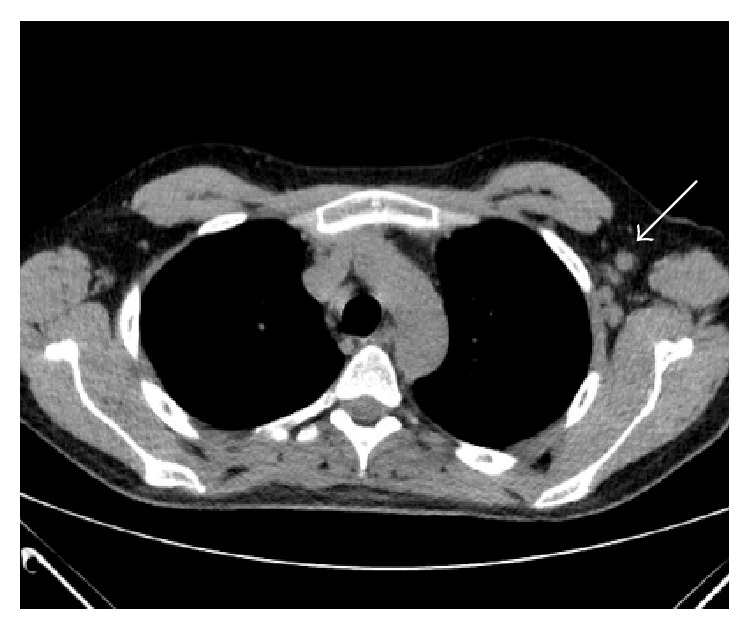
CT scan of thorax. CT of chest showing enlarged axillary nodes (arrow).
